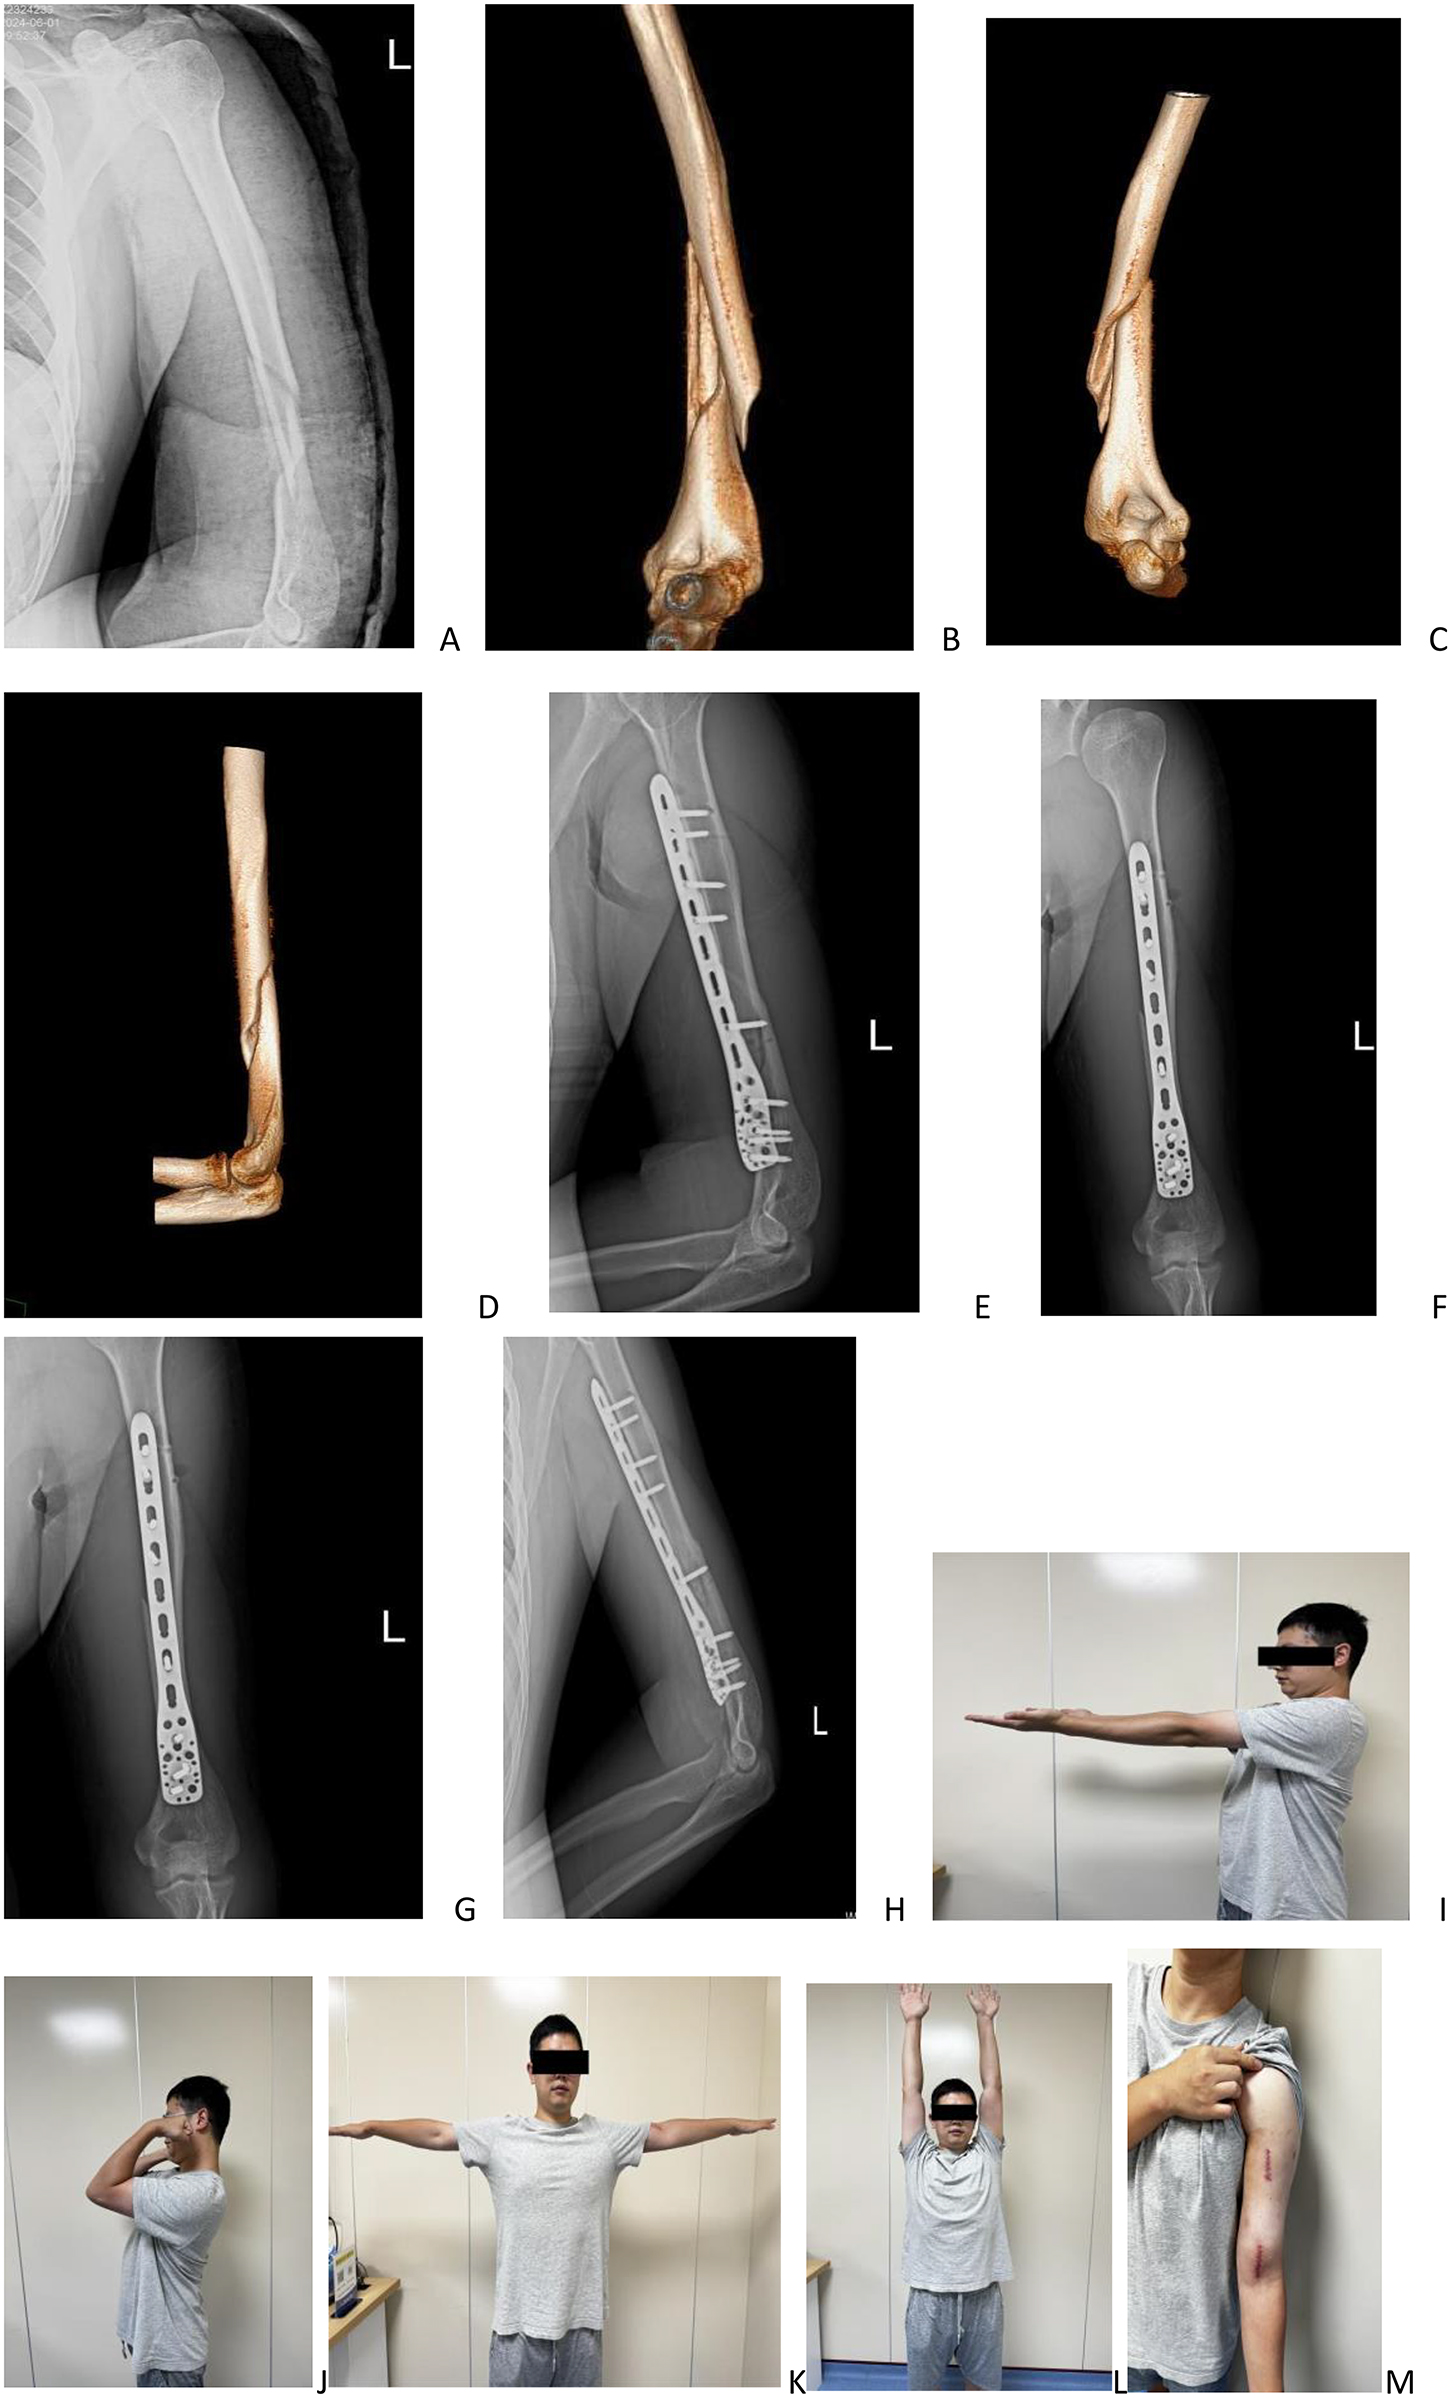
(Patient A): a 33-year-old male, presented with pain in his left upper arm following a fall. Radiographic evaluation, including X-ray and CT imaging, confirmed a fracture involving the distal segments of the left humerus (A-D). The fracture was treated using an inverted PHILOS plate fixation via an anterior minimally invasive surgical approach (E-F). A follow-up X-ray taken two months postoperatively demonstrated satisfactory fracture healing (G-H). At follow-up, the patient’s left elbow exhibited a functional range of motion from 0° to 130°. The patient achieved full functional recovery, as reflected by a score of 100 on both the Mayo Elbow Performance Score (MEPS) and the Constant-Murley shoulder score (I-L). The total length of the surgical incisions was approximately 15 cm, and the incisions healed without complications (M)
